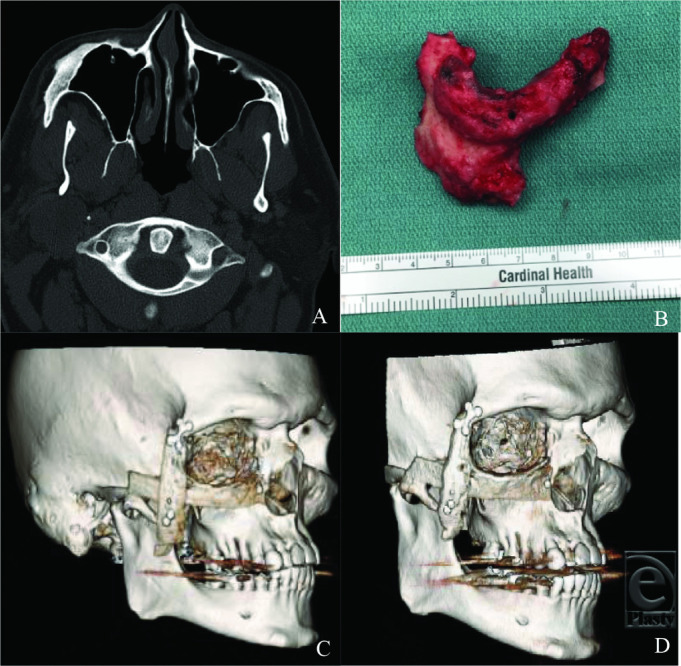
(*a*) Preoperative CT scan with right malar thickening from fibrous dysplasia. (*b*) Resected lesion including the right zygomatic arch, zygoma, inferior and lateral orbital wall, and lateral maxilla. (*c*) 3D maxillofacial CT on postoperative day 1. (*d*) 3D maxillofacial CT scan 1 year postoperatively. CT indicates computed tomography; 3D, three-dimensional.
